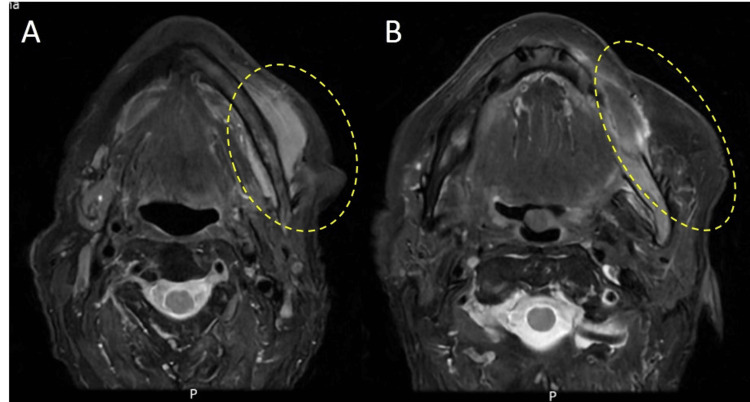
Axial and coronal T2-weighted MRI demonstrating an infiltrative lesion of the left hemimandible. (A, B) Axial T2-weighted images showing a hypointense, diffuse infiltrative lesion involving the left hemimandible (yellow dashed circles), with soft tissue extension and preservation of the cortical outline.
